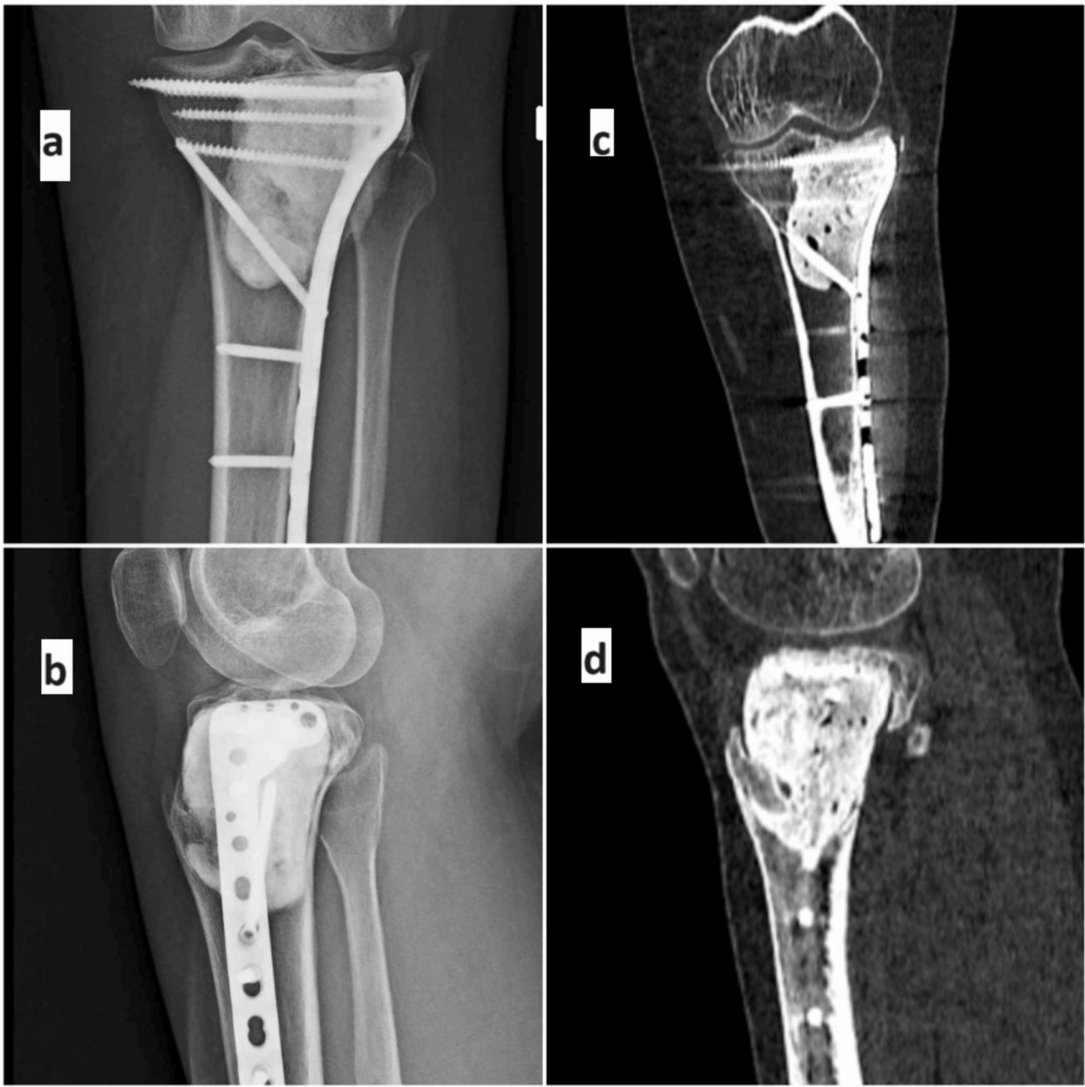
Follow-up radiographs and CT scan Three-year follow-up imaging (a, b: plain radiographs, anteroposterior, and lateral projections, respectively, showing anatomically contoured articular surface. c, d: CT coronal and sagittal images showing well-incorporated graft and smooth articular surface.) CT: computed tomography
